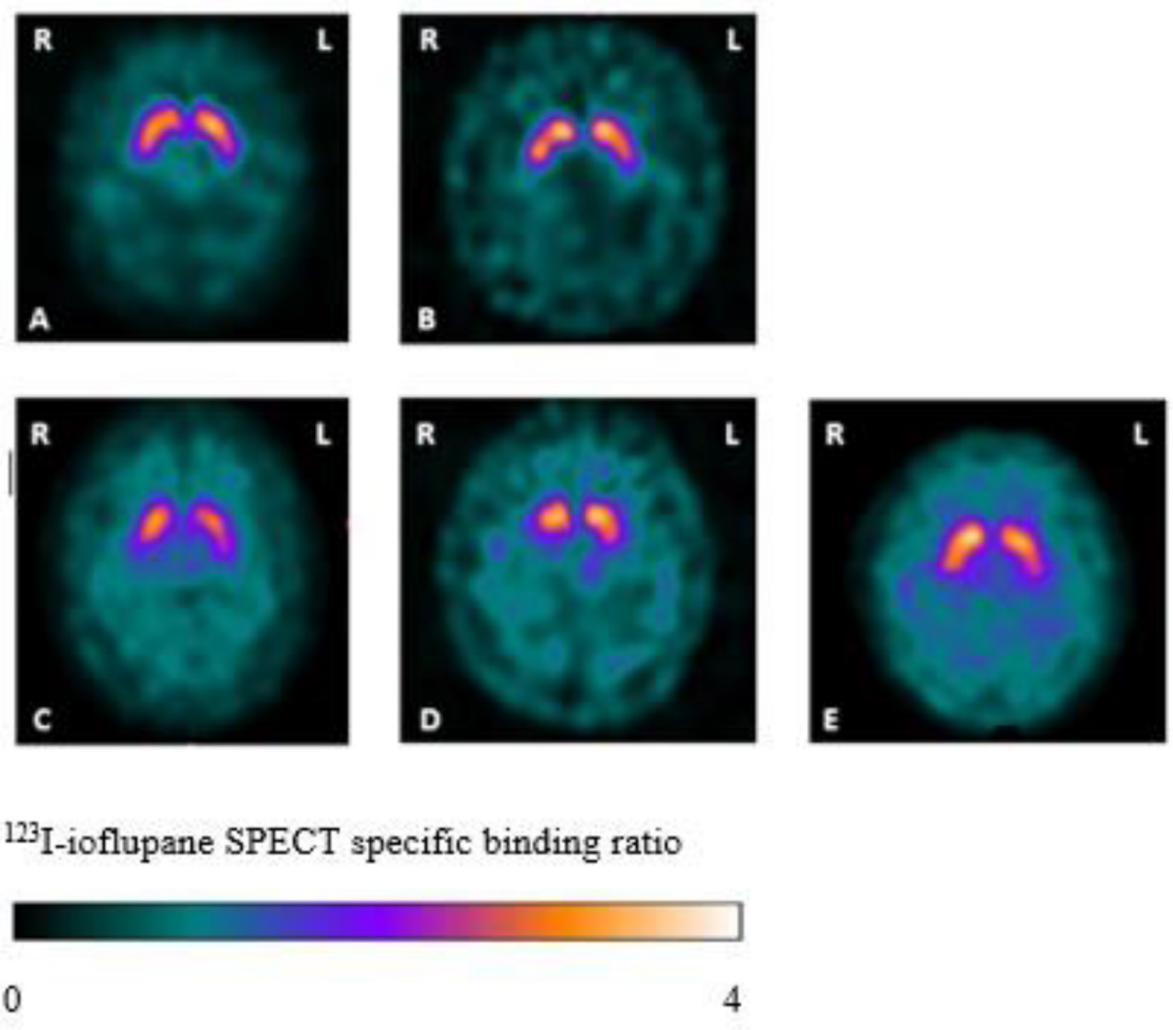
Examples of ^123^I-ioflupane SPECTs from the study and their visual ratings (transversal SPECT slices at the level of the striatum). (A) Normal. (B) Equivocal. (C) Abnormal type 1. (D) Abnormal type 2. (E) Balanced striatal loss. L, left side; R, right side.
